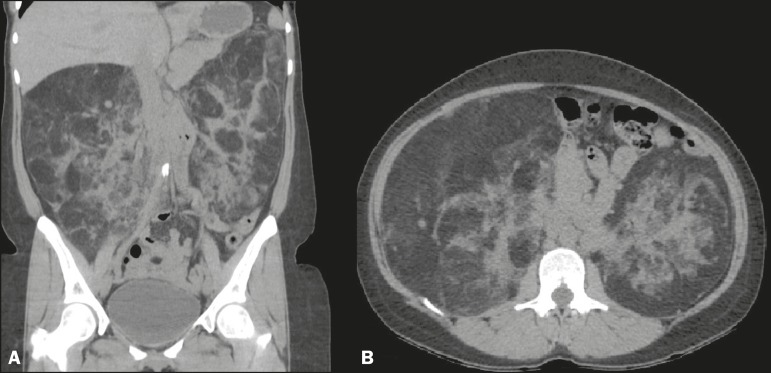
Non-contrast-enhanced CT of the abdomen with coronal reconstruction
